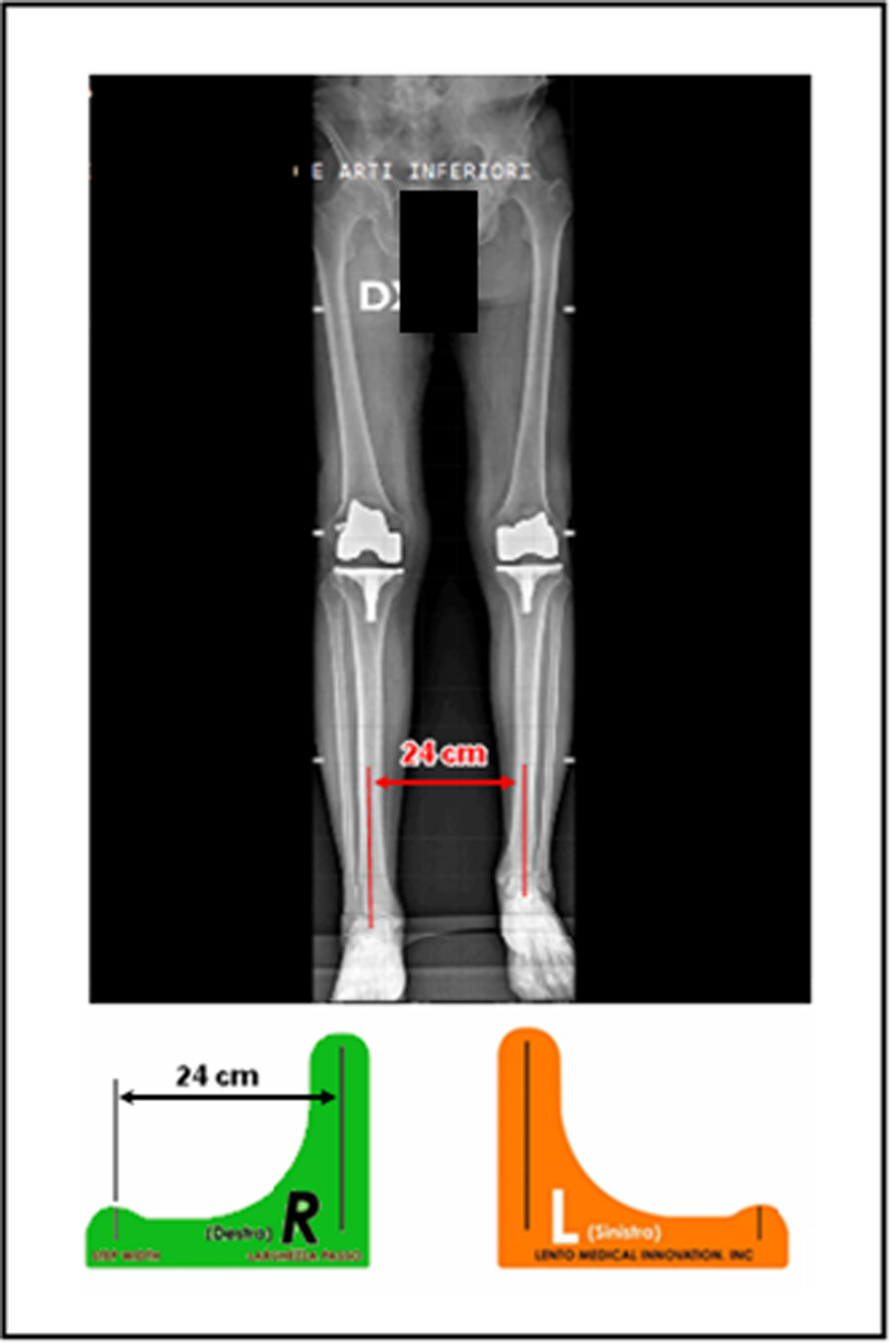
Step width of 24 cm and long leg post‐op radiograph.
